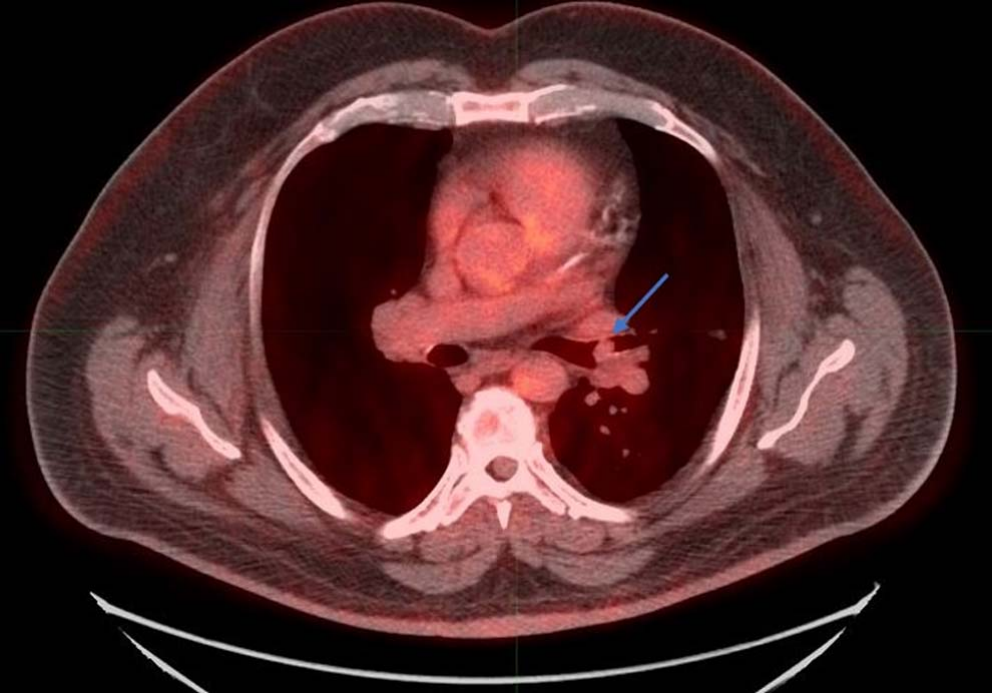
Fused F18-fludeoxyglucose positron emission tomography-computed tomography axial image shows the left upper lobe endobronchial lesion without significant radiotracer uptake, suggesting a low-grade, well-differentiated tumor (arrow).
